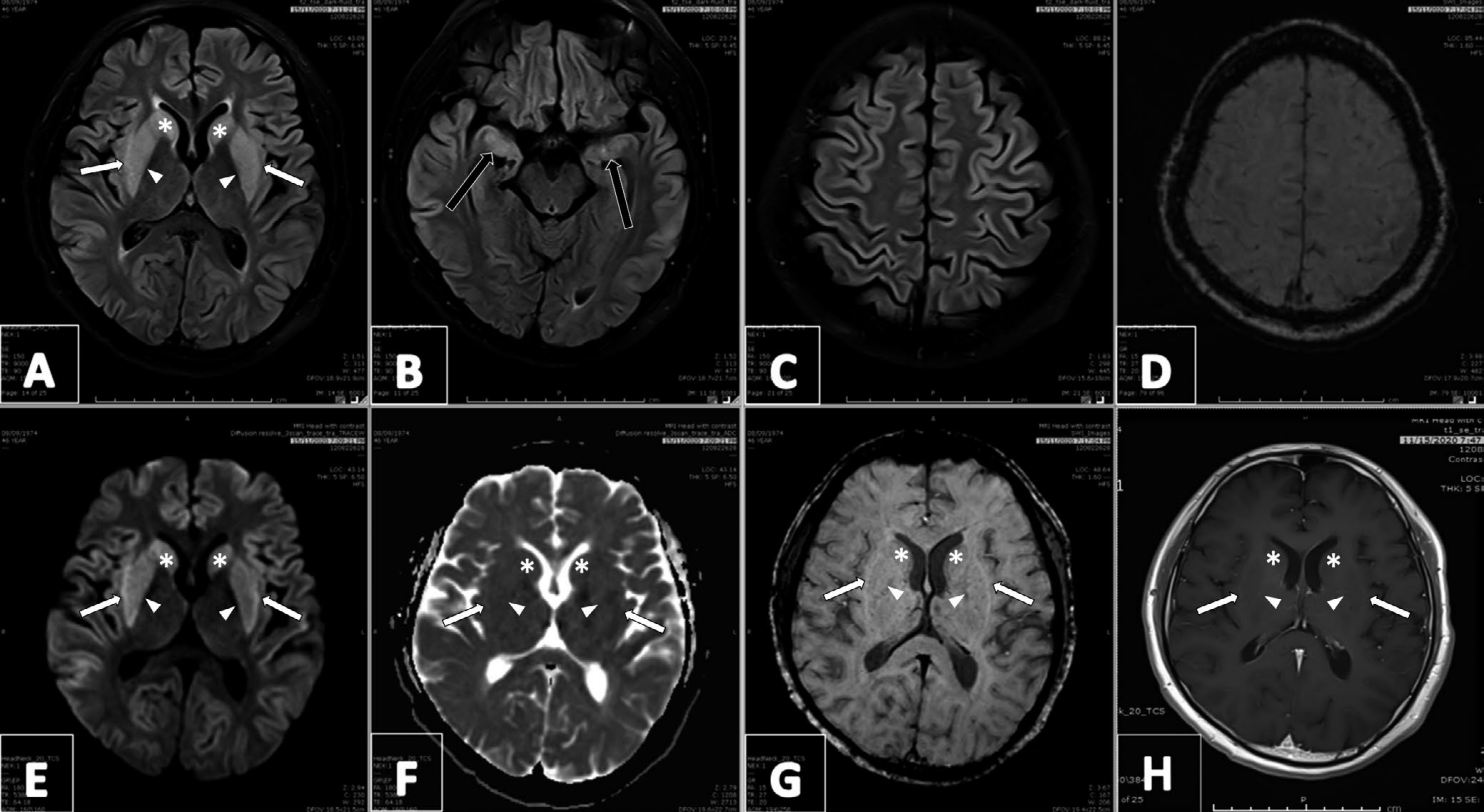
Magnetic Resonance Imaging (MRI) of the brain. High signal intensity on T2 FLAIR (A) and DWI (E), while hypointense signal on T1‐weighted images (H) along with corresponding low signal on ADC map (F) signify edema and restricted diffusion due to osmotic demyelination. Notice bilateral amygdala hyperintensity (black arrow in B) along with cortical gray matter swelling (C), showing COVID‐19‐related encephalopathic changes. No SWI changes (D, G). Symbols point out the involved regions, that is, caudate nuclei (asterisk), putamen (arrowhead), and external capsule (white arrow)
